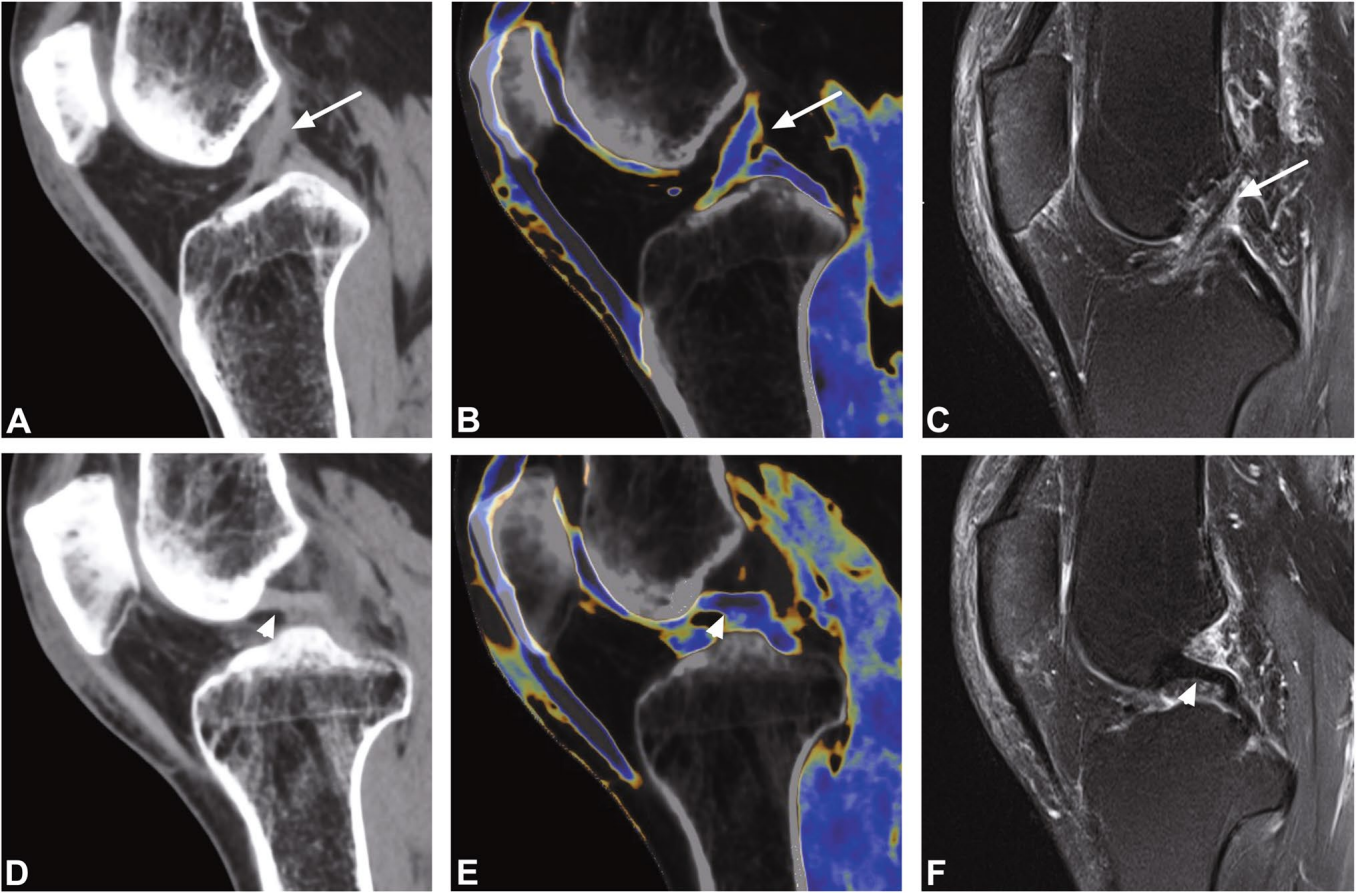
Standard sagittal unenhanced grayscale CT (A, D), color-coded collagen reconstructions (B, E), and unenhanced proton density-weighted MRI series with fat saturation (C, F) of a 28-year-old patient with acute knee pain following a bicycle accident demonstrate intact anterior (arrows) and posterior cruciate ligaments (arrowheads). The collagen signal is visualized blue by using default postprocessing settings
